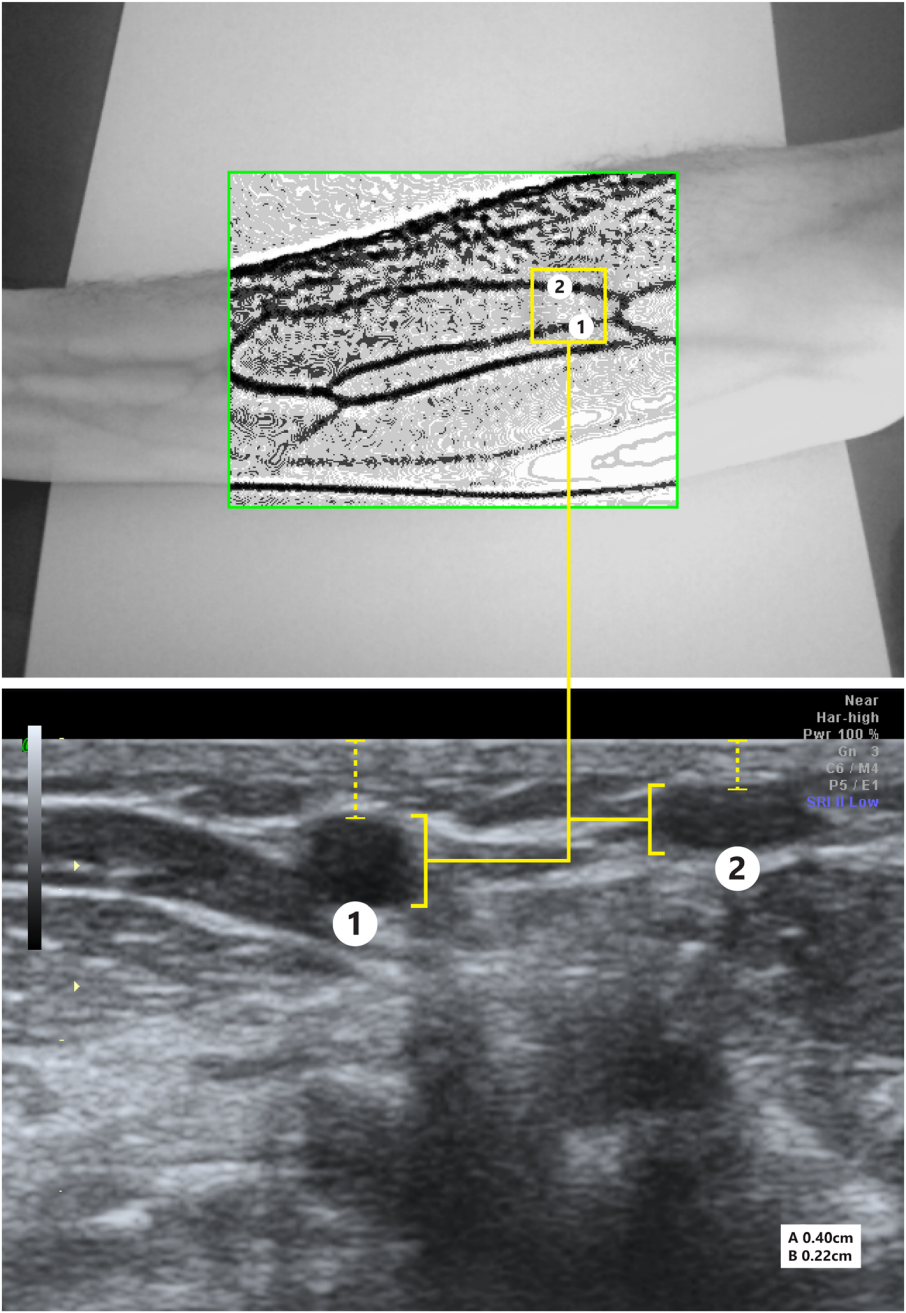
An image from the prototype (above) and an associated cross-sectional ultrasound image confirming the presence of a pair of veins below the skin surface. A (0.40 cm) and B (0.22 cm) represent the depth of the veins as identified with the ultrasound device.
